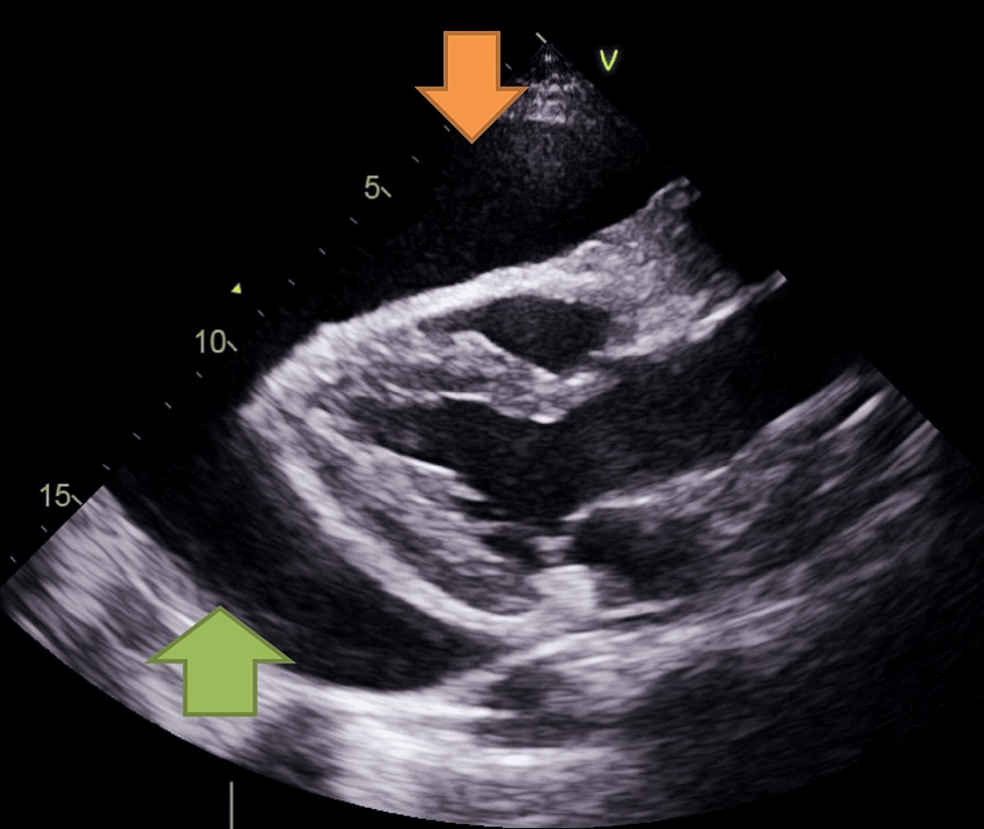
Echocardiography parasternal long-axis view showing large pericardial effusion (pointed coloured arrows)
